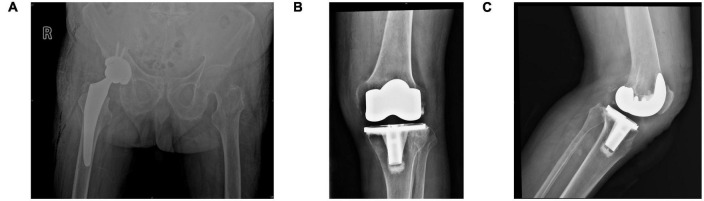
(A) Bedside hip radiograph (anteroposterior view) obtained the day following surgery showing anteversion and abduction angles within the normal range based on the Lewinnek and Callanan safe zones. (B,C) Radiographs of the knee (anteroposterior and lateral views) obtained the day following TKA showing correct prosthesis placement. TKA, total knee arthroplasty.
